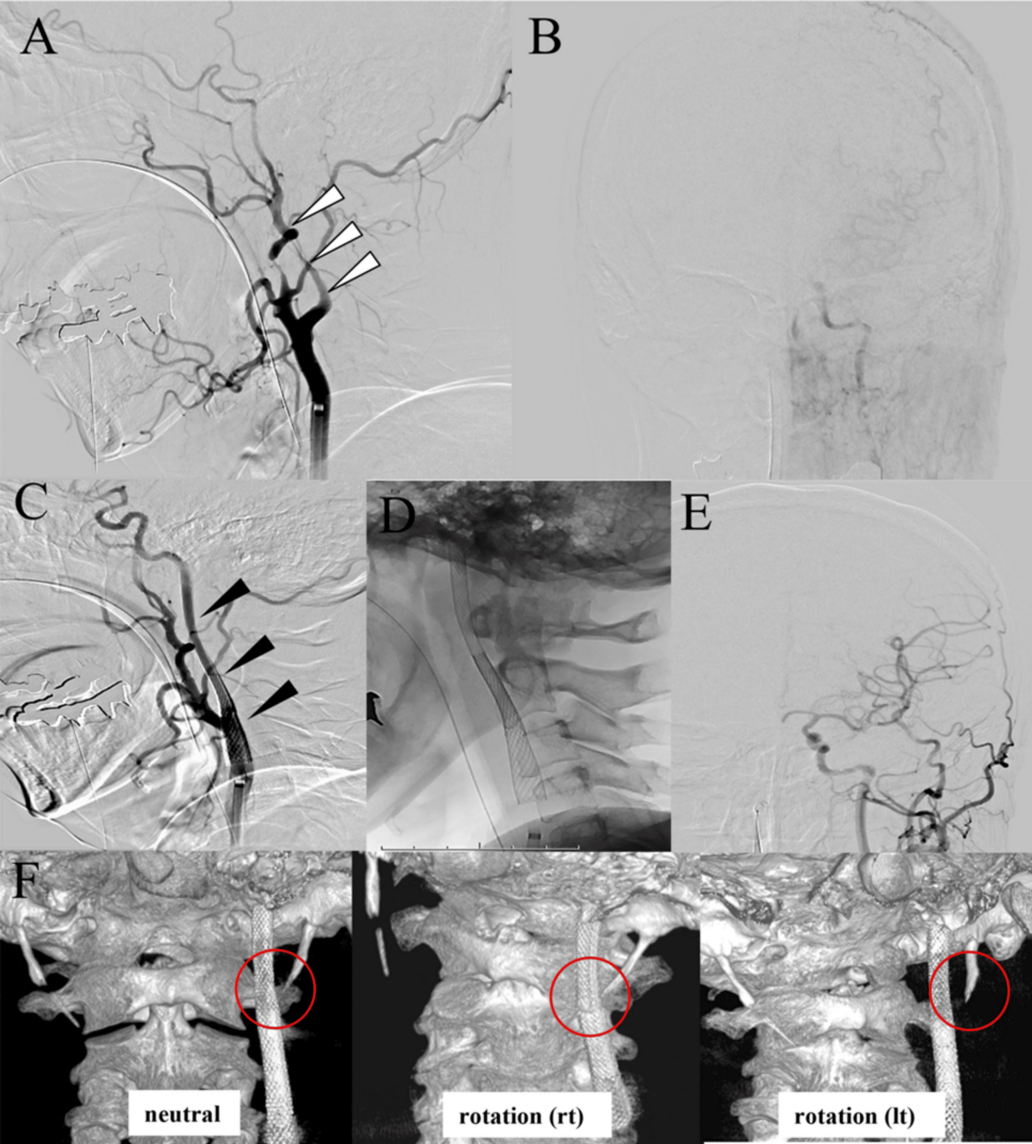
Digital subtraction angiography and cone-beam CT with dynamic head rotation during the initial intervention. Preoperative images showing near occlusion of the left ICA (A) with reduced blood flow in the left MCA territory (B). Postoperative images demonstrating successful CAS with restored patency of the left ICA (C), the stent shadow visible in the non-subtracted image (D), and improved perfusion in the left MCA territory (E). Cone-beam CT in dynamic head positions (left: neutral, center: rightward rotation, right: leftward rotation), revealing a close and variable spatial relationship between the left SP and ICA with head rotation (highlighted in red circles) (F). Note that the SP is observed to directly impinge on the ICA at rightward rotation. CAS: carotid artery stenting; ICA: internal carotid artery; MCA: middle cerebral artery; SP: styloid process
